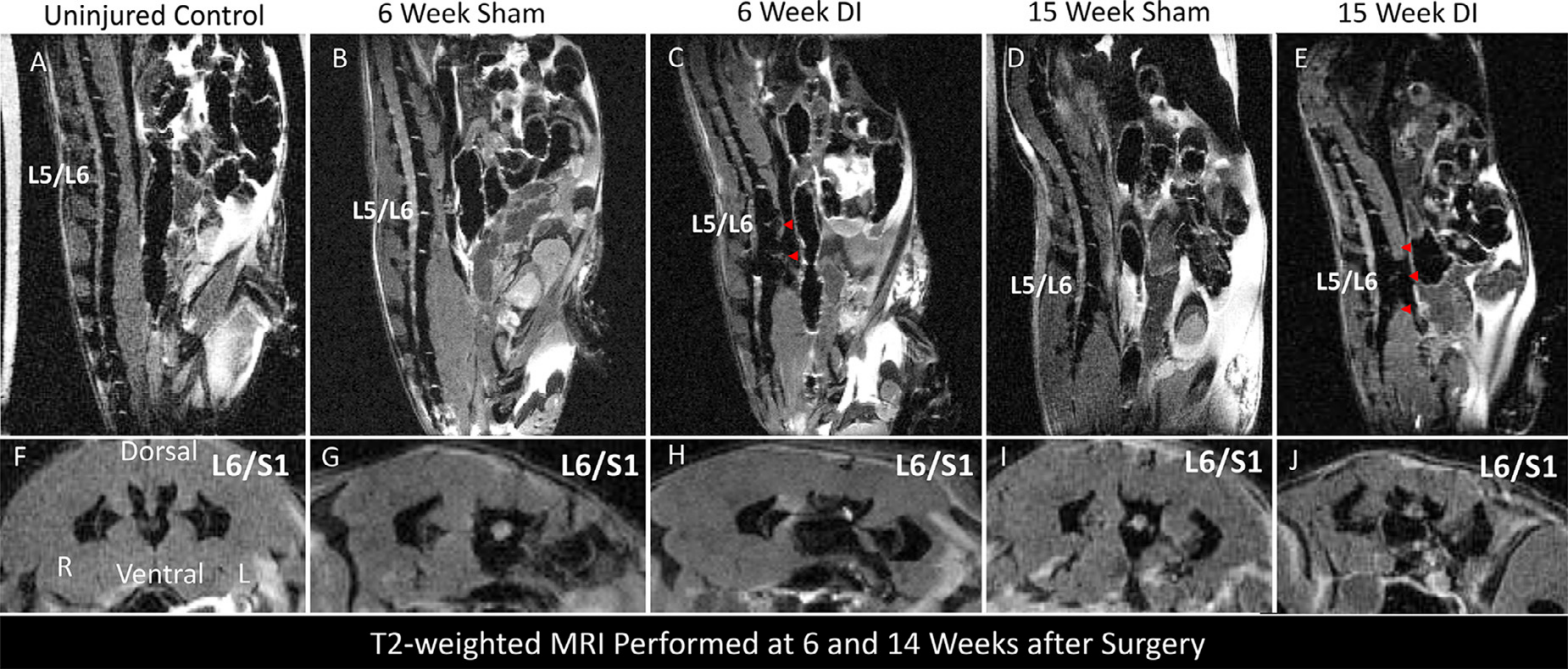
Disc injury results in progressive degenerative changes in the lumbar spine at 6 and 15 weeks. Representative T2-weighted sagittal (A-E) and axial (F-J) MRI images at 6 and 15 weeks obtained post-mortem using 14T MRI. Red arrows on sagittal images indicate severe disc degeneration characterized by loss of bright signal seen at uninjured levels.
